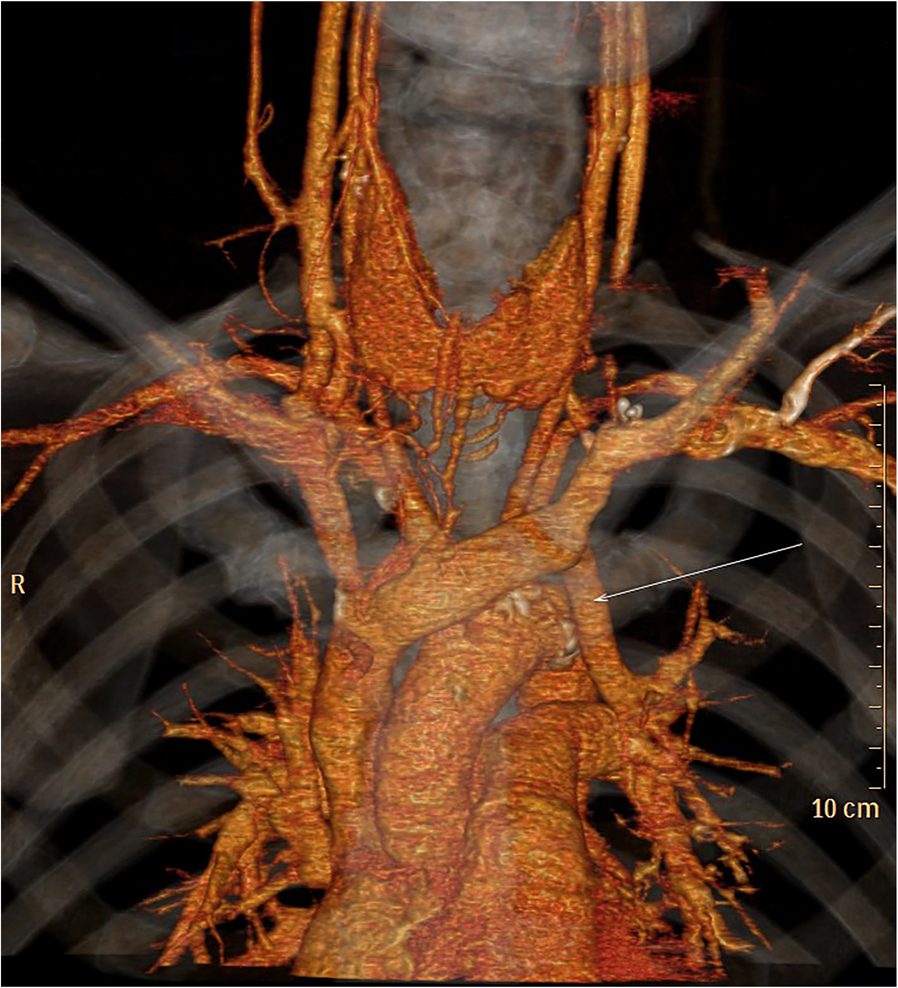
CTA of central vein and three-dimensional reconstruction confirmed PLSVC which connected with pulmonary vein
